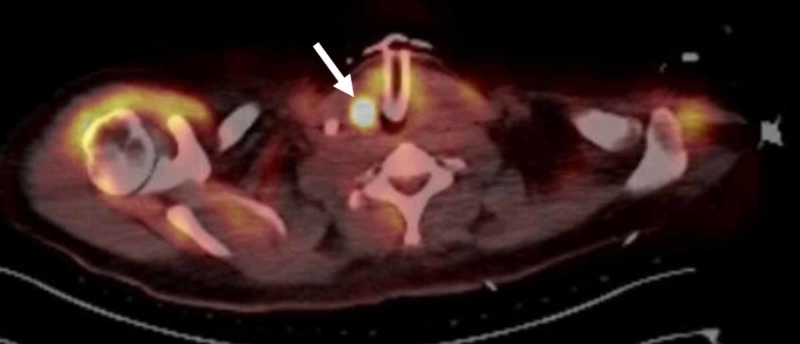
FDG-PET scan showing intensely hypermetabolic right thyroid nodule (white arrow) not detected on prior imaging scans to evaluate for occult malignancy. FDG-PET: Fluorodeoxyglucose positron emission tomography
